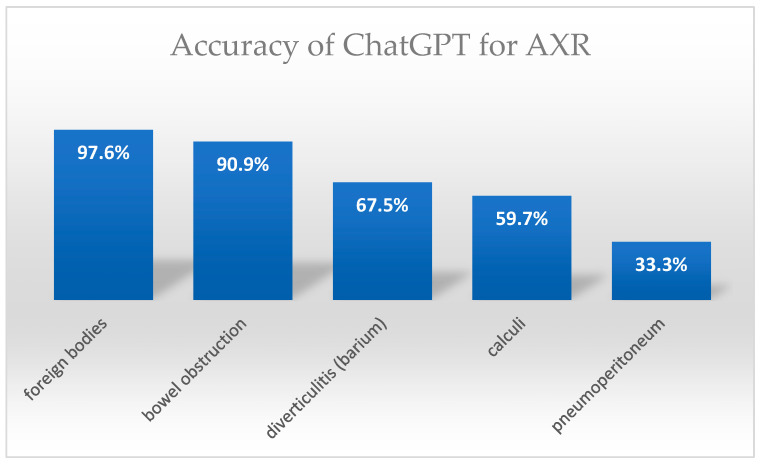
Accuracy of ChatGPT in diagnosing different abdominal X-ray (AXR) pathologies. AXR performance was highest for intestinal obstruction and foreign bodies but weaker for pneumoperitoneum, renal calculi, and diverticulitis.
